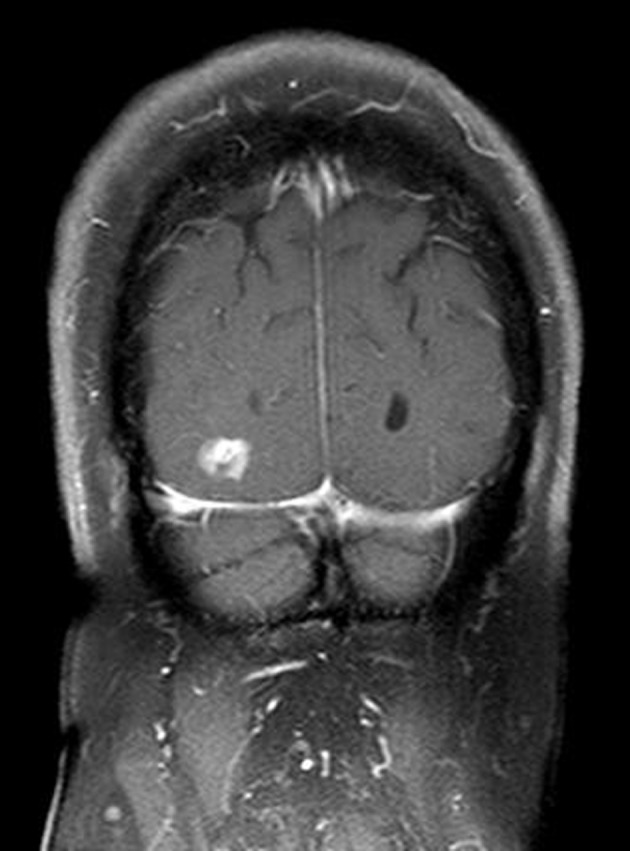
MRI of brain with and without gadolinium-based contrast demonstrating contrast enhancing lesion within the right occipital lobe.
